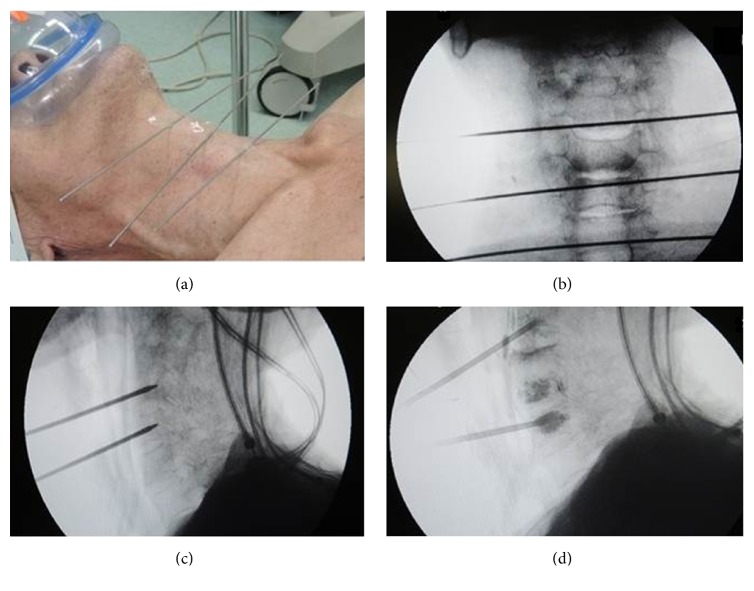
(a) PVP was performed using anterolateral approach with all patients in supine position under general anesthesia or local anesthesia. (b) The target vertebra was located under the guidance of a C-arm digitalized X-ray machine. (c) Confirm the corrected position of the needle. (d) The injection was stopped when the cement filled up the lesion or any paraspinal leakage was observed.
